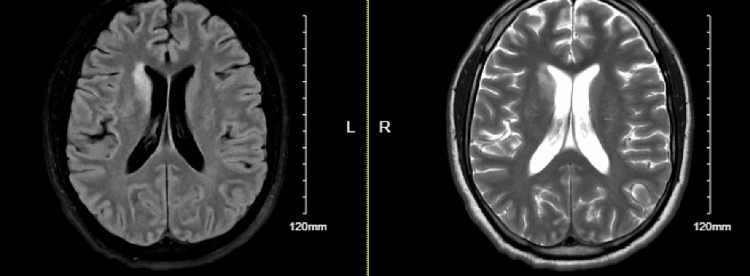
Day 4 MRI non-contrast Radiologic interpretation of increased hyperintensities in both ADC and DWI along the bilateral corona radiata right anterior caudate nucleus, dorsal left putamen and bilateral cerebellar hemispheres with an exception of a small area in the caudate. These imaging findings are consistent with acute or subacute interstitial disease. Differential includes inflammatory/post inflammatory, infectious/postinfectious, autoimmune, and PRES or demyelinating processes. DWI: Diffusion-weighted imaging; ADC: Apparent diffusion coefficient; PRES: Posterior reversible encephalopathy syndrome
